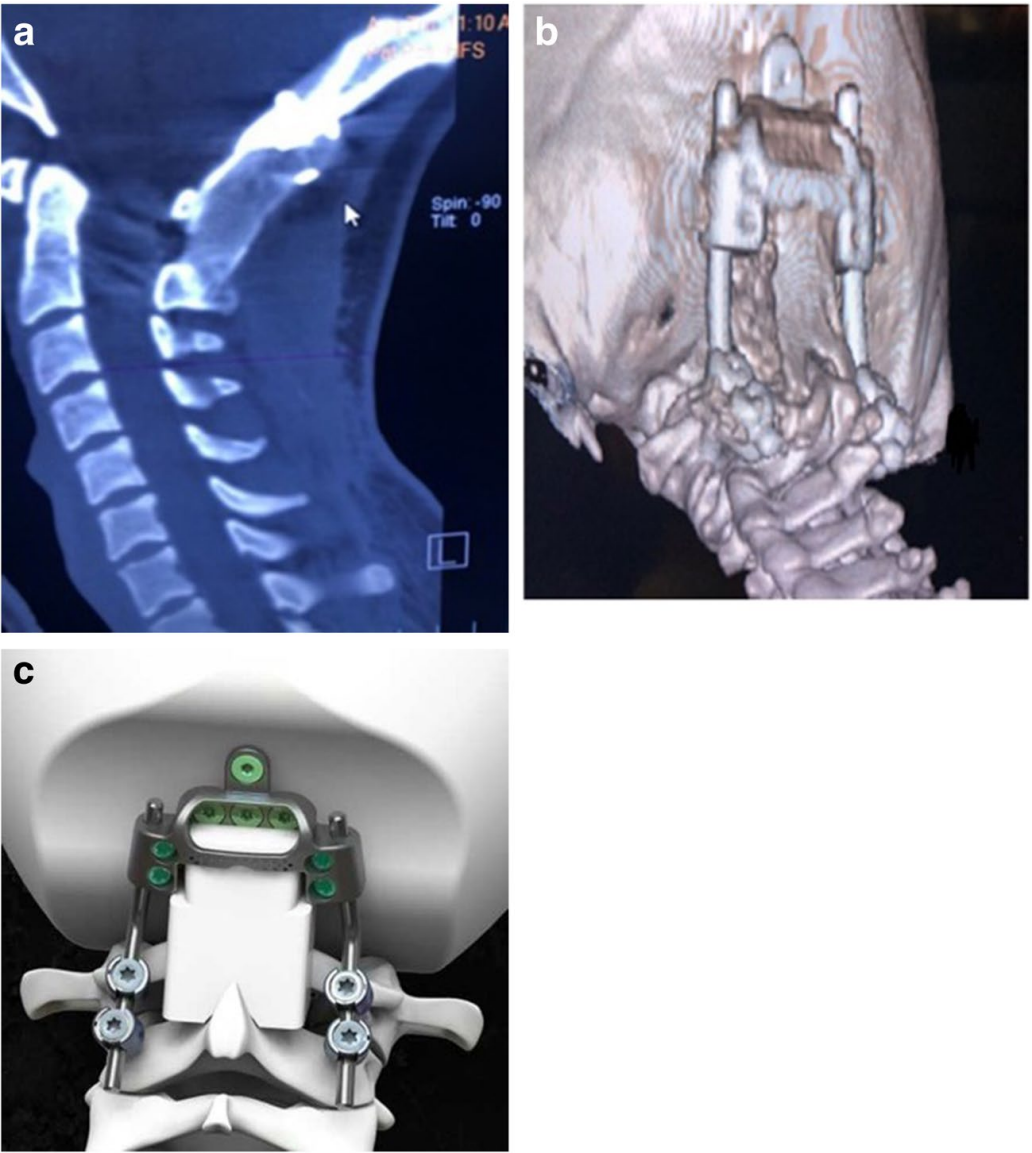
a CT scan, mid-sagittal view of the craniocervical junction showing the occipito-cervical fusion/stabilization (OCF). The bone allograft inserts superiorly into the aperture of the suboccipital plate. It lies against the occiput superiorly, the C1 posterior arch, and is notched inferiorly to encompass C2 spinous process and lamina. b CT scan, 3D reconstruction, showing the carefully tapered bone graft as it encompasses the occiput superiorly and lamina and C2 spinous process inferiorly. c The bone graft is secured within the aperture at the base of the CCI device (Cranio-Cervical Integration device, CCI®, LifeSpine Inc., Huntley, IL). The device has a smooth contour and small footprint to maximize bone surface area for fusion
